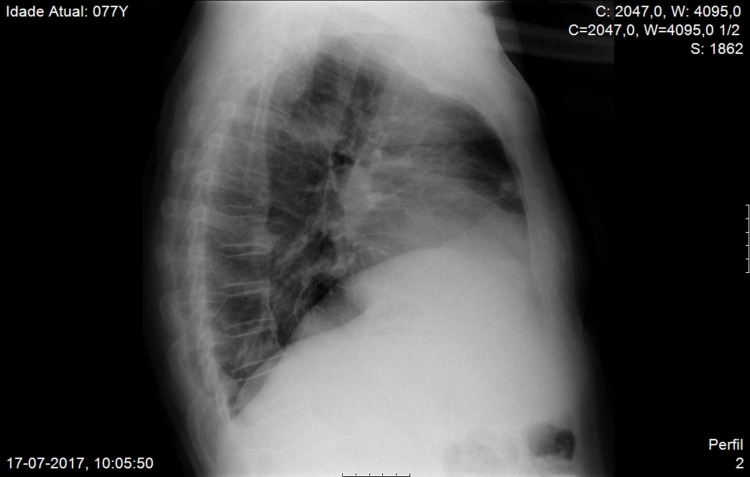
Chest X-ray, lateral view Chest X-ray, lateral view, on the first admission, with residual aspects of organized cryptogenic pneumonia of the right lung the patient had the year before
